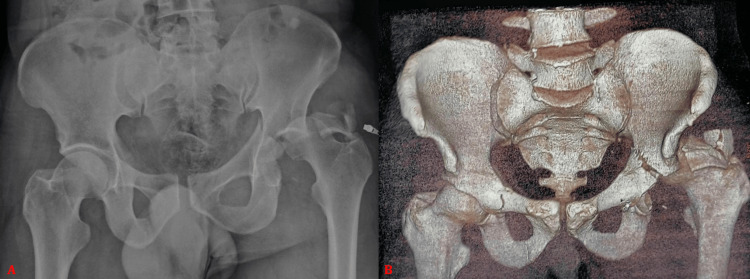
Anteroposterior pelvic radiograph and (B) three-dimensional CT bone reconstruction showing a transverse acetabular fracture with posterior wall involvement according to the Judet and Letournel classification, associated with a posterosuperior dislocation of the femoral head in a 46-year-old male patient.
